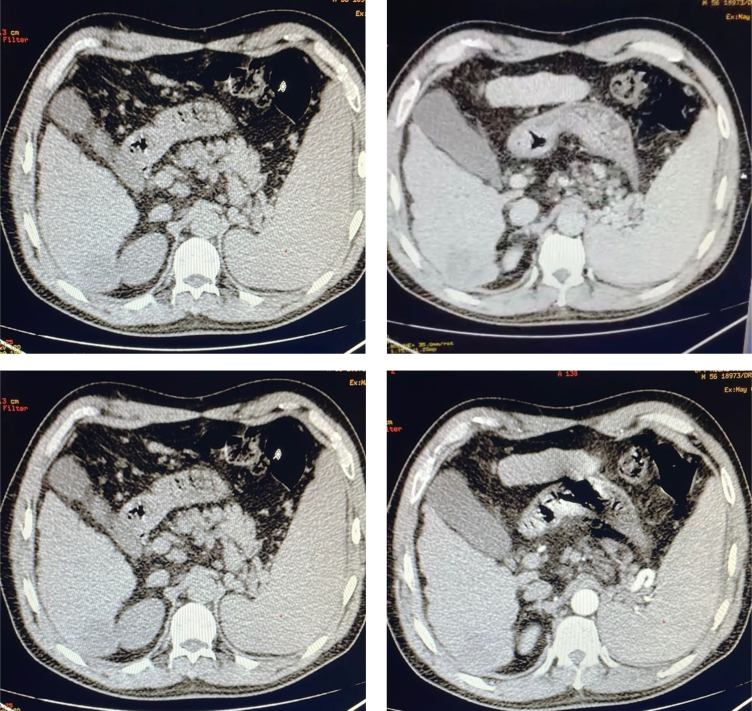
The CT scan images at various arterial and venous phases: a dysmorphic liver with cirrhosis and a spontaneously hypodense lesion in segment VI, measuring 44×33 mm, with heterogeneous enhancement in the arterial phase and homogenized in the venous phase.
